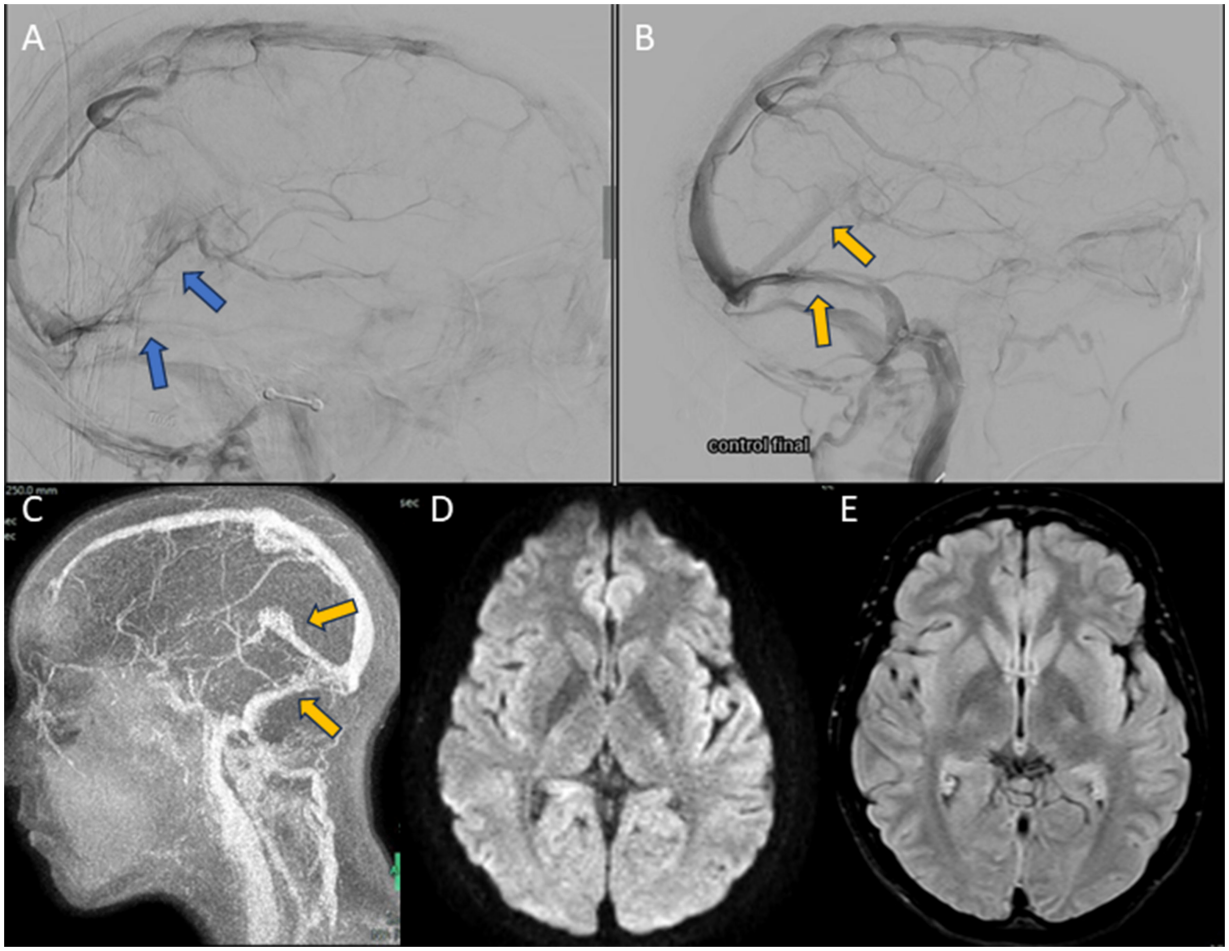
Images obtained during the course of case 2. (A, B) Selective right internal carotid arteriography in venous phase, lateral views. (A) Pre-MT image. Thrombosis of rectus sinus, deep cerebral veins, transverse and sigmoid sinuses (blue arrows) is seen. (B) Post-MT image. Recanalization of the rectus sinus (yellow arrow), torcula, superior sagittal sinus, and both transverse sinuses (yellow arrow) is seen. (C–E) Brain MRI performed 7 days after MT. (C) 2D-TOF MRI angiography, sagittal view. Shows the complete recovery of the venous system (yellow arrow). (D) DWI sequence. No acute ischaemic lesions are evident. (E) FLAIR sequence. No images suggestive of residual ischaemic or haemorrhagic pathology are seen.
